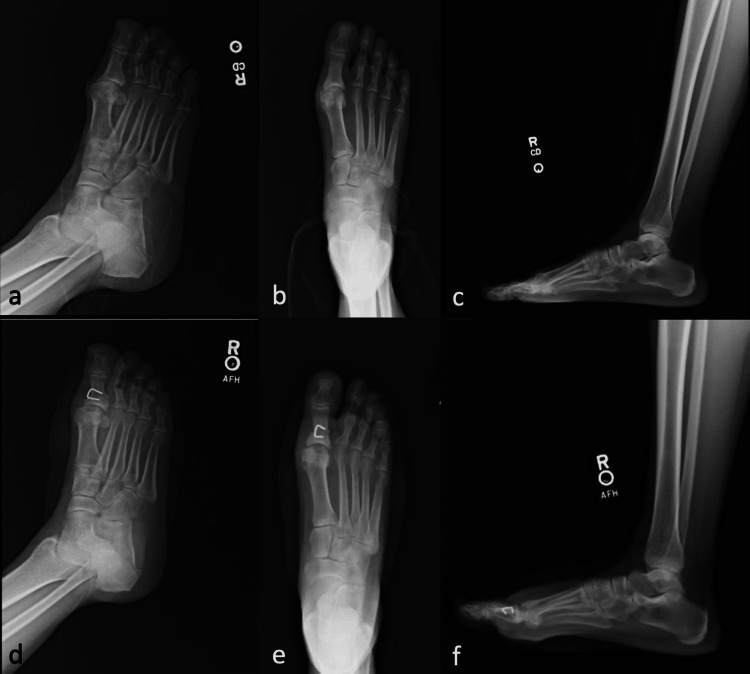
Preoperative oblique (A), anteroposterior (B), and lateral (C) weight-bearing radiographic views of the right foot with hallux rigidus compared with postoperative oblique (D), anteroposterior (E), and lateral (F) weight-bearing views of the right foot following Synthetic Cartilage Implant placement and Akin osteotomy.
